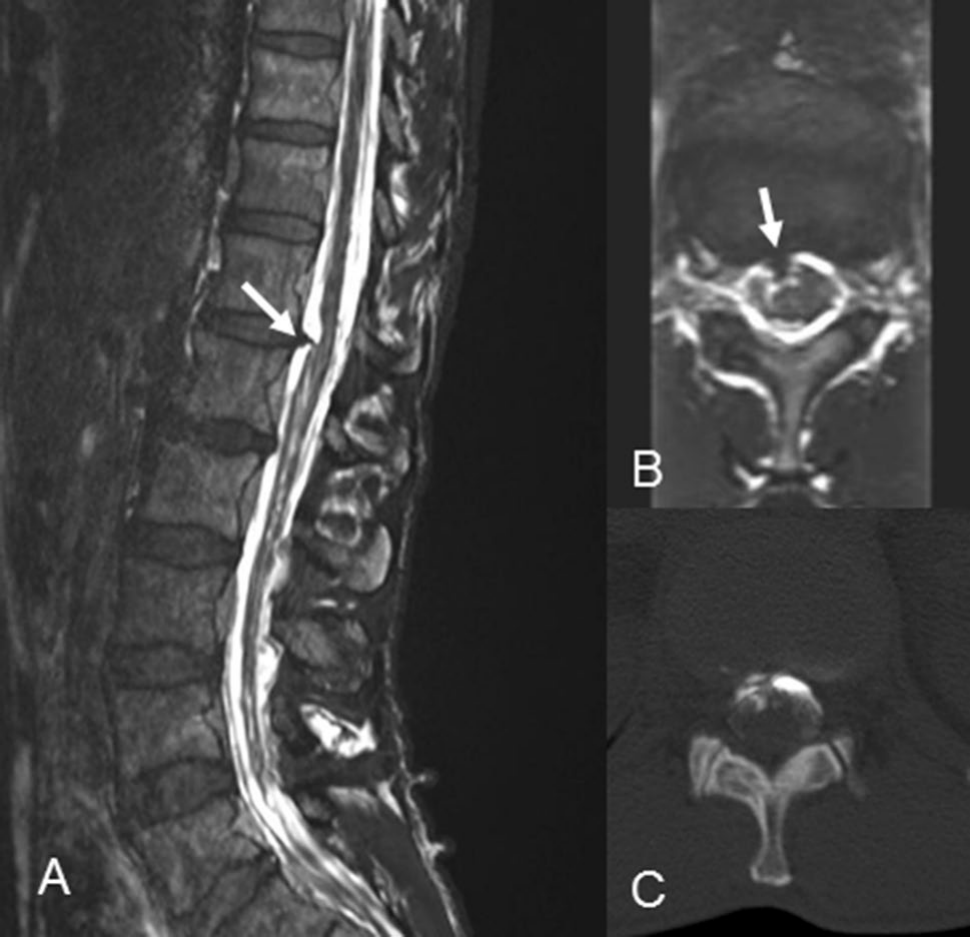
a, b Sagittal and axial CISS MRI shows the discogenic spur at T12/L1(arrow); c CT-myelography with epidural contrast-pooling and right-sided ventromedial contrast agent extravasation
